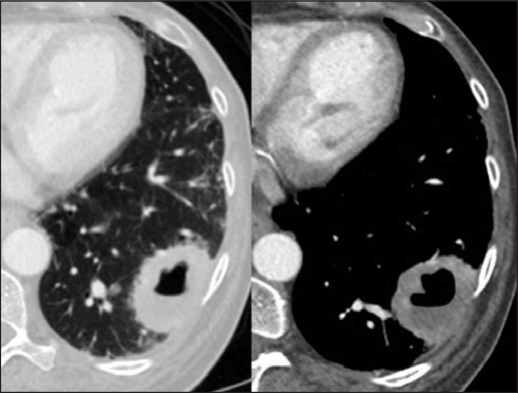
Lung cancer.* A 65-year-old female patient with a smoking history of 50 years was admitted with hemoptysis and malaise, no fever. CT showed a large cavitary lesion with varying wall thickness. Note that without the clinical history this case is impossible to differentiate from the case presented in Figure 1.
